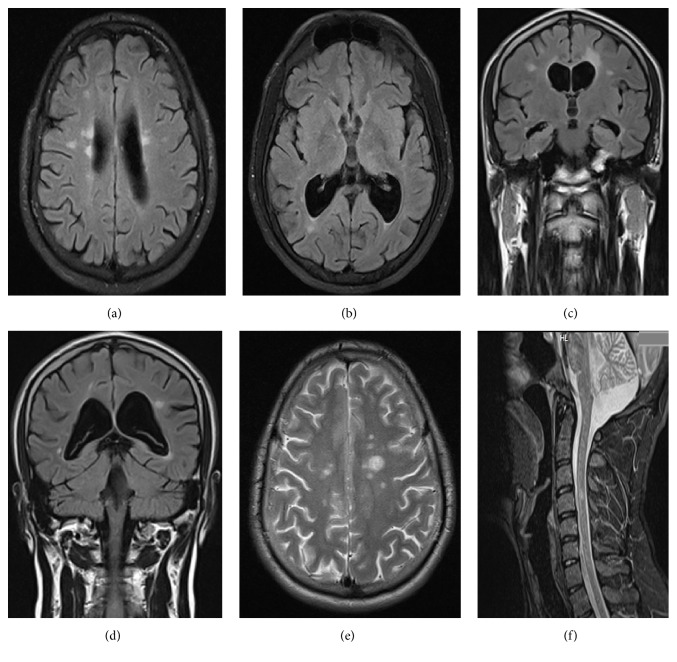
(a, b, c, and d) Flair MRI slices (axial and coronal slices) show periventricular and juxtacortical high intensity lesions. (e) Axial T2 MRI shows multiple juxtacortical high intensity lesions. (f) Sagittal T2 MRI of the cervical spinal cord shows T2 hyperintense lesion at the level of C4.
